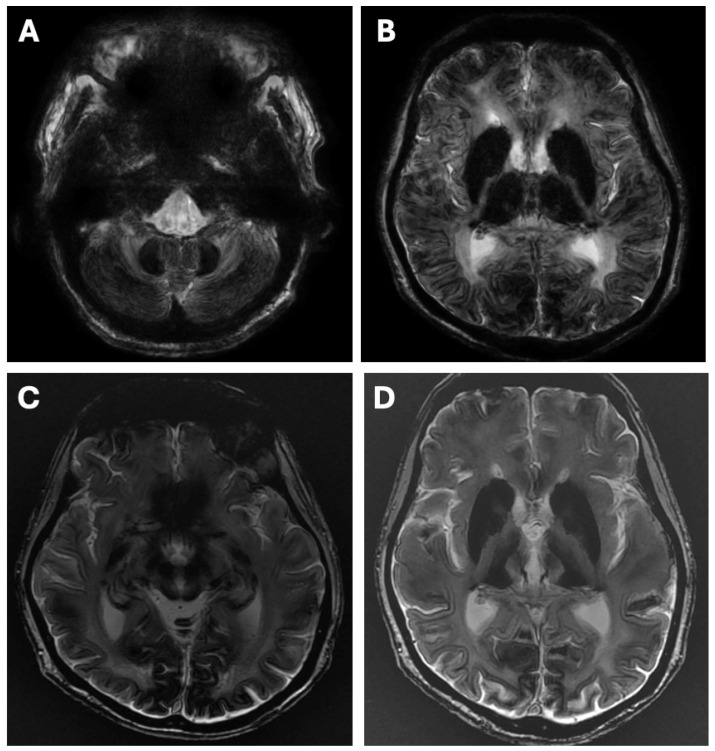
Brain MRI pathology in aceruloplasminemia—symmetrical hypointense signal in caudate, globus pallidus, putamen, thalamus, red nucleus, dentate in susceptibility-weighted imaging (SWI) sequences (A,B) and T2* sequences (C,D) (authors’ material from the Department of Neurology).
